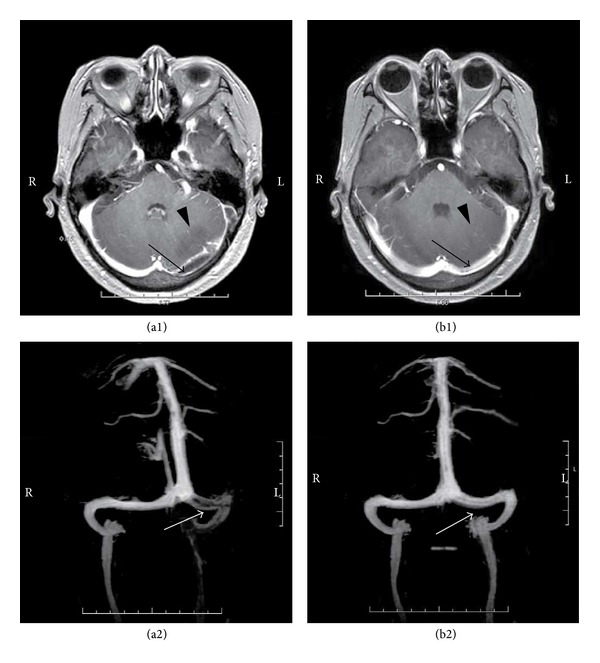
Brain MRI and MRV scan before and after treatment. MRV scan demonstrates loss of signal in the left transverse and sigmoid sinus, representing venous sinus thrombosis before treatment (indicated by arrows on (a1) and (a2)). The signal in the left transverse and sigmoid sinus recovered two months after the initiation of treatment (indicated by arrows on (b1) and (b2)), representing recanalization. MR image shows a patchy hypointense lesion in the left hemisphere of the cerebellum before treatment (indicated by arrow head on (a1)), and this patchy hypointense lesion recovered in the corresponding area after treatment (indicated by arrow head on b1).
